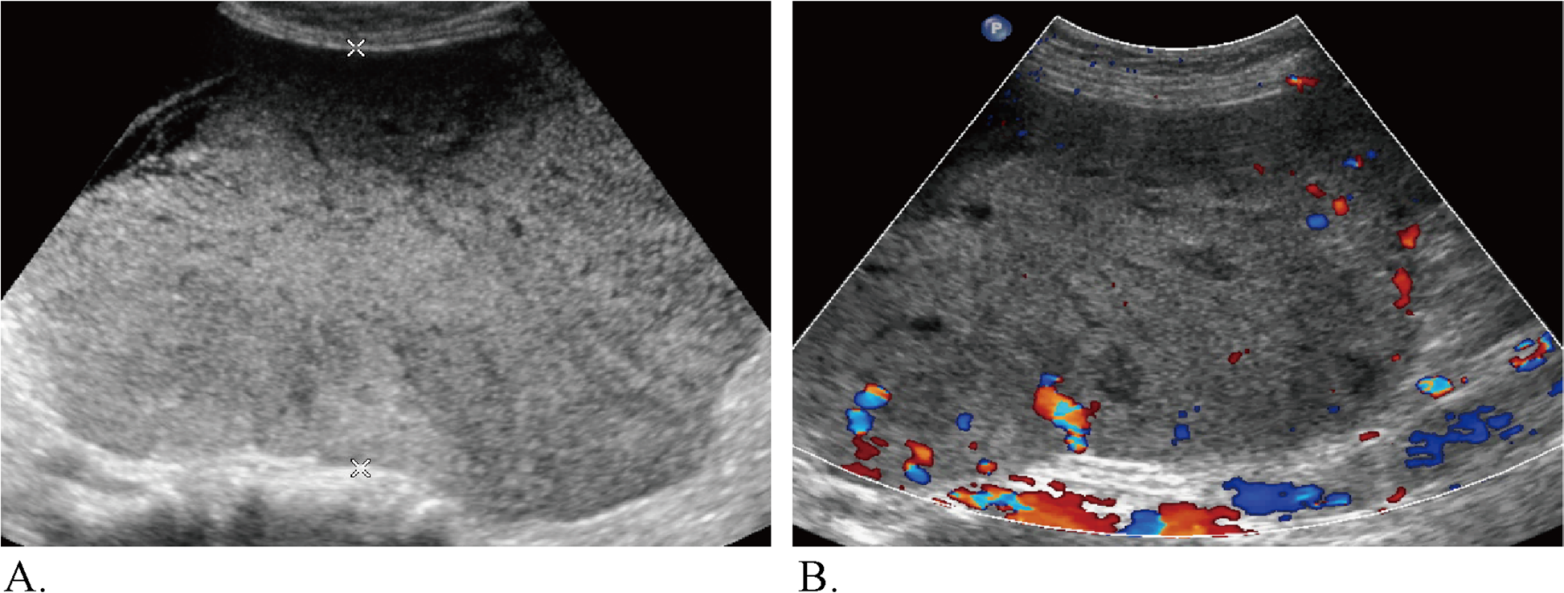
Ultrasound images of a 53-year-old woman whose CA 125 level was 965 U/ml and who had a pathologically proven endometrioid carcinoma. A Abdominal grayscale ultrasound showed a 17.6-cm irregular solid tumor component in the right adnexa. B A color Doppler ultrasound image showing moderate flow (color score = 3). The lesion was categorized as IOTA SR M1 and O-RADS 5, according to the sonographic findings
